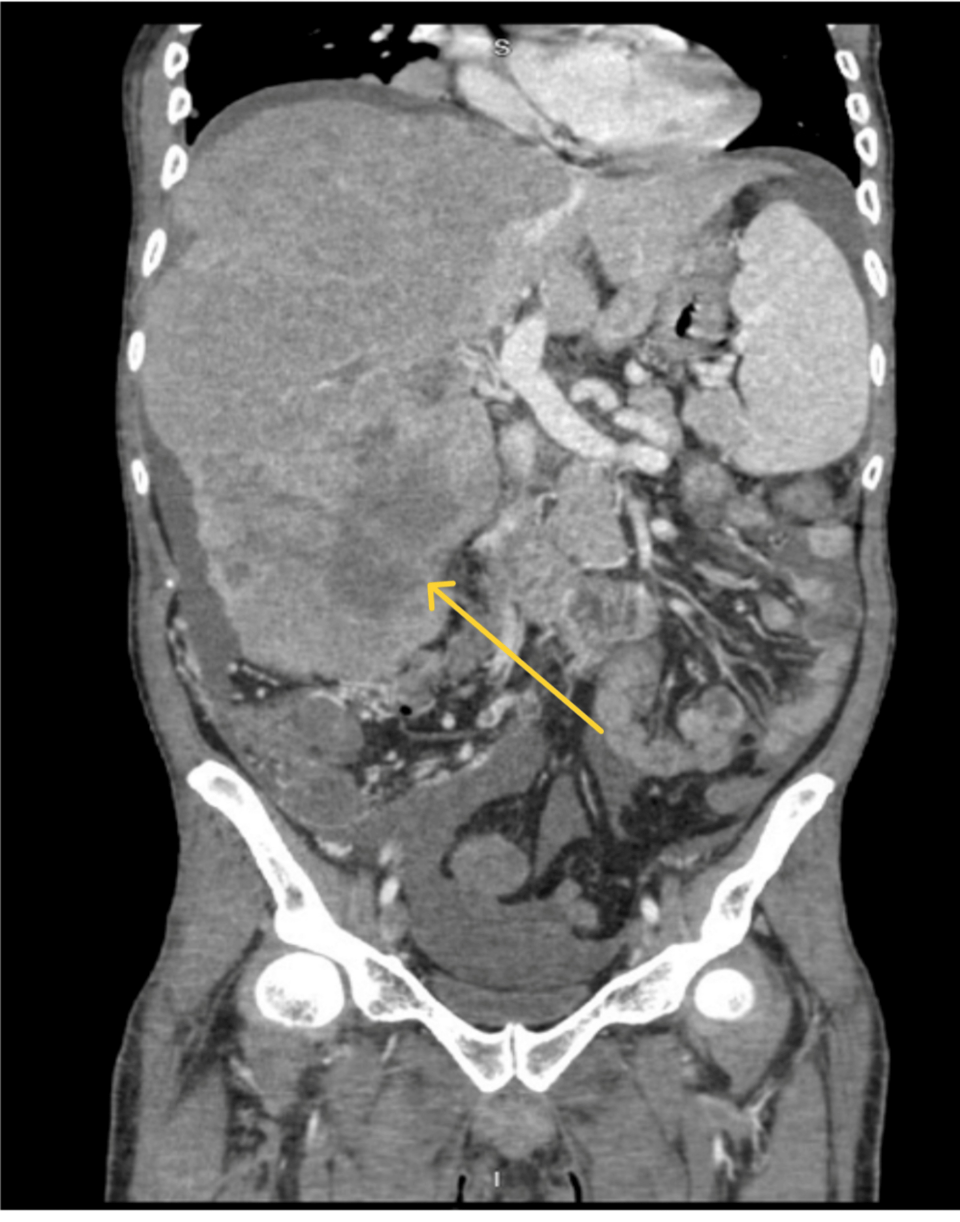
Computed tomography (CT) scan of the abdomen, coronal view showing enlarged liver with liver lesions (yellow arrow)
